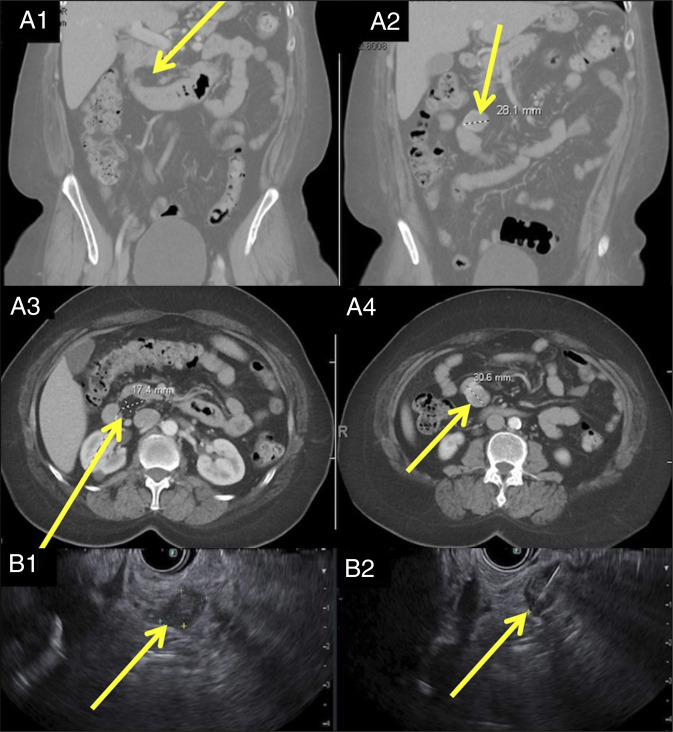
Cross-sectional imaging and endoscopic ultrasound. Computed tomography abdomen/pelvis with several nonobstructing small bowel masses visualized, concerning for gastrointestinal stromal tumors associated with neurofibromatosis 1 (A1–A4). Endoscopic ultrasound with a mucosal lesion (B1) in the duodenum and an intramural (subepithelial) lesion (B2) in the duodenum that appeared to originate from within the muscularis propria.
